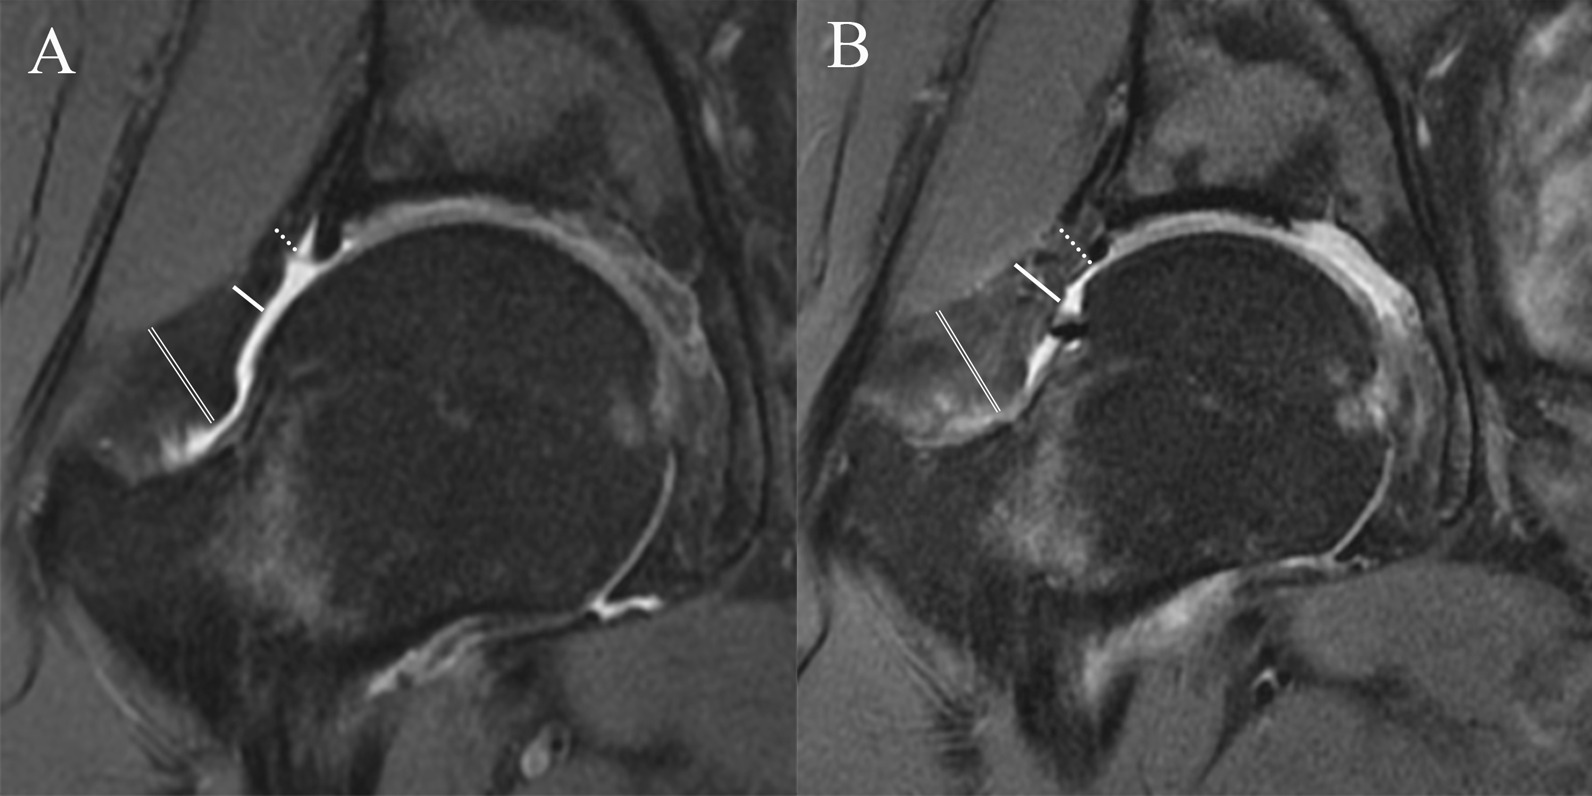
A Preoperative measurements of capsular thickness at the proximal (dotted line), middle (single solid line), and distal (double line) site. B Measurements of capsular thickness at the proximal (dotted line), middle (single solid line), and distal (double line) site in the same patient in MRI follow-up
